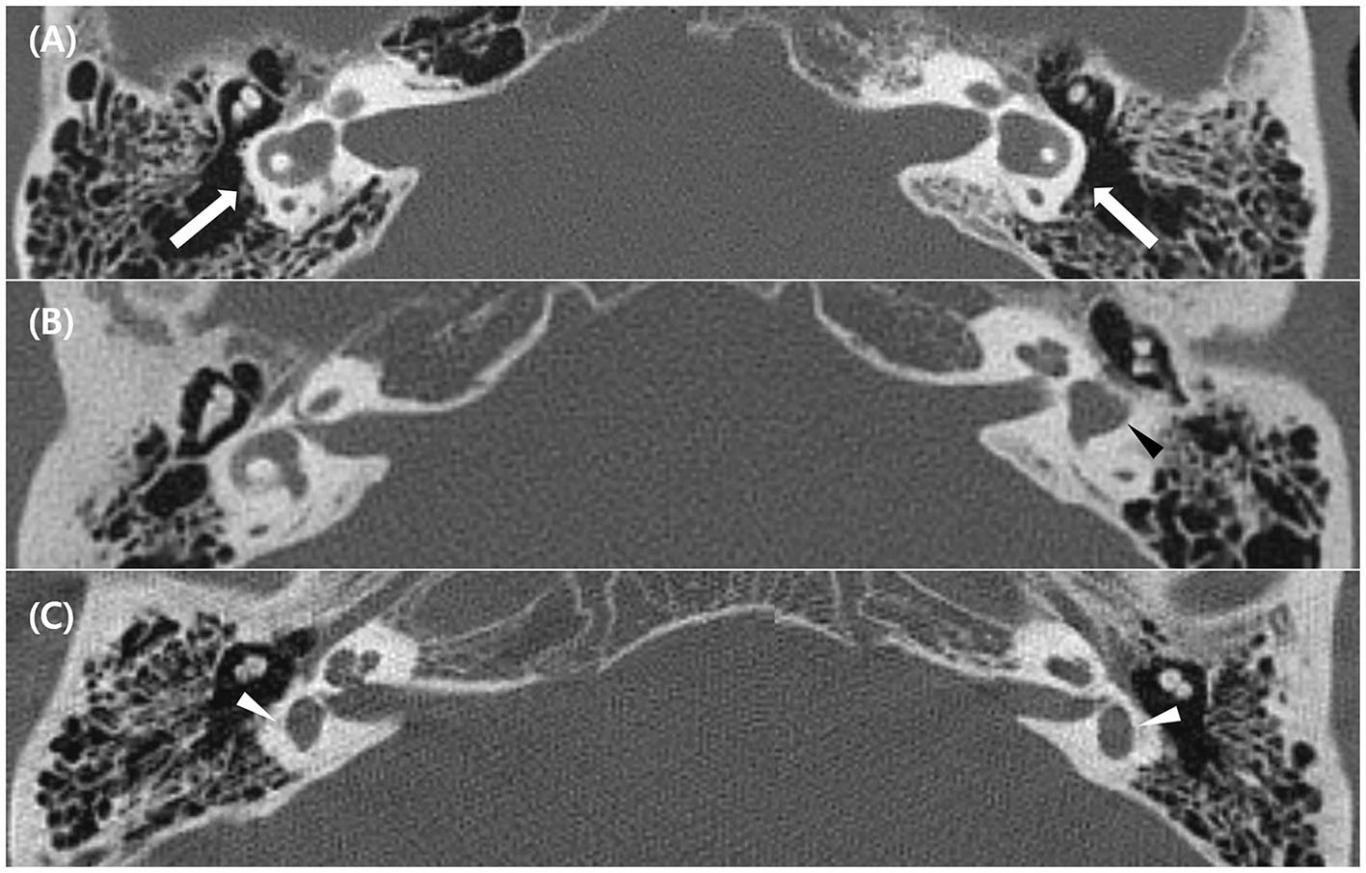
Temporal bone computed tomography (TBCT) image of the lateral semicircular canal (LSCC) dysplasia. (A) Bilateral LSCC hypoplasia with dilated SCC and enlargement of the vestibule (white arrows) (Patient 2 in Table 1). (B) Unilateral LSCC hypoplasia without a central bony island (black arrowhead) (Patient 8 in Table 1). (C) Bilateral LSCC aplasia with enlarged vestibule only (white arrowheads) (Patient 4 in Table 1).
